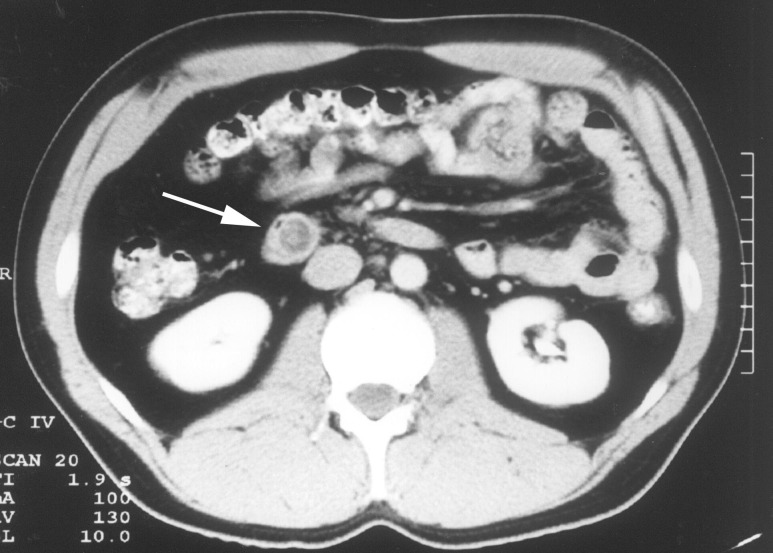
Abdominal computed tomography (CT) scan demonstrates an ampullary mass
